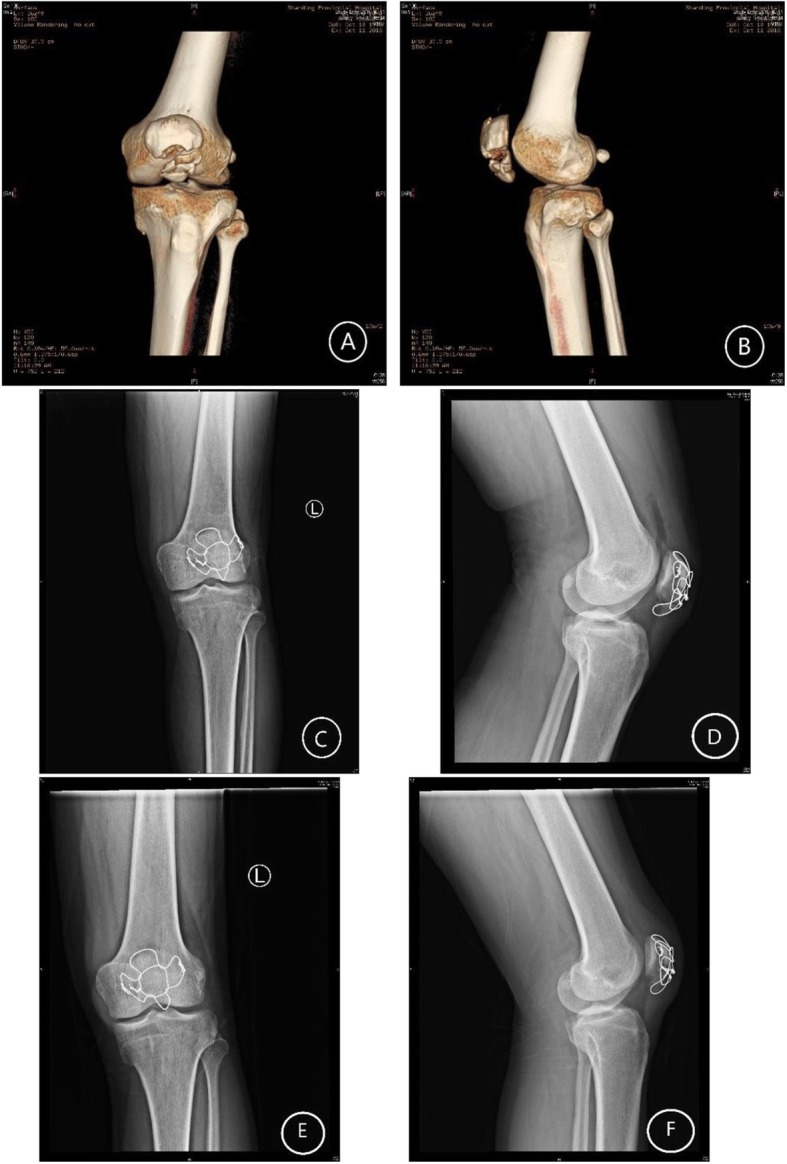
A 39-year-old male with comminuted patellar fracture. a, b CT before surgery. c, d Post-fixation with modified cerclage wiring. e, f 3 months after surgery
